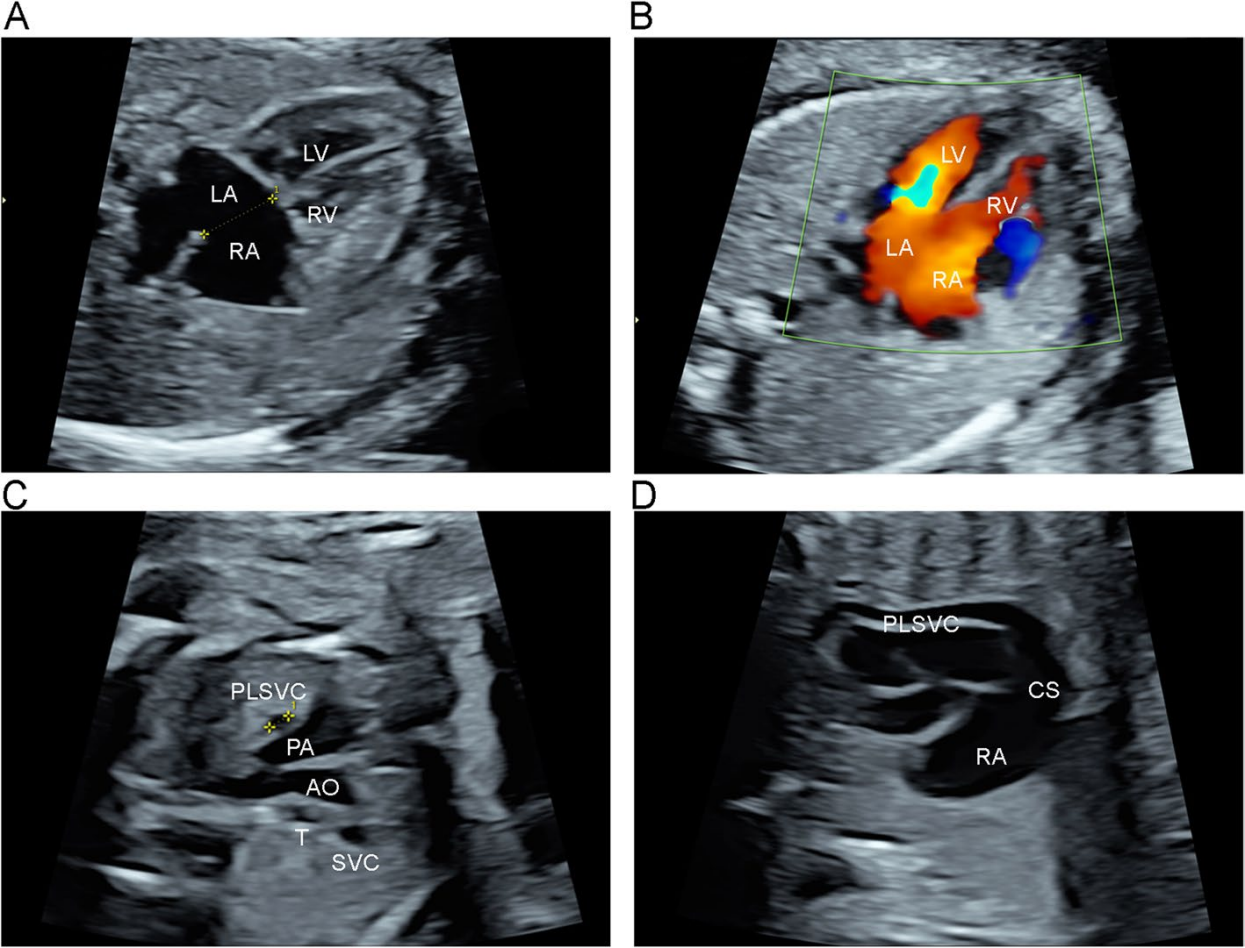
The results of prenatal ultrasound. A The primary atrial septal defect and (B) the communication of blood between the left and right atrium. C The vascular shadow on the left side of the pulmonary artery and (D) merge into the dilated coronary sinus. LA left atrium; RA, right atrium; LV, left ventricle; RV, right ventricle; PLSVC, perpetual left superior vena cava; PA, pulmonary artery; AO, aorta; T, trachea; SVC, superior vena cava
